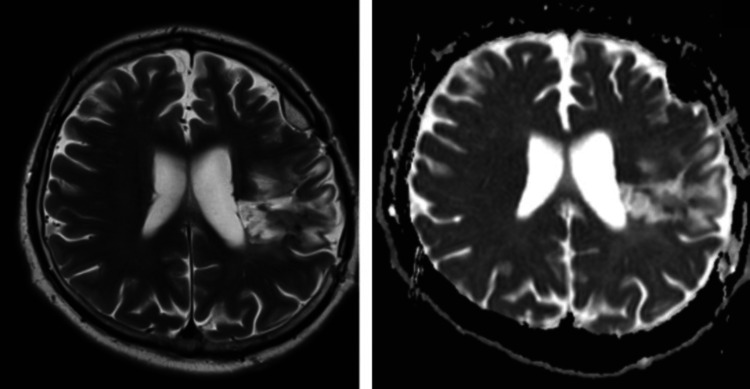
Brain MRI at the one-month follow-up T2-weighted imaging (left) and diffusion-weighted imaging (right) show a sequelae ischemic area located in the motor area of the frontal lobe, anterior to the central sulcus.
